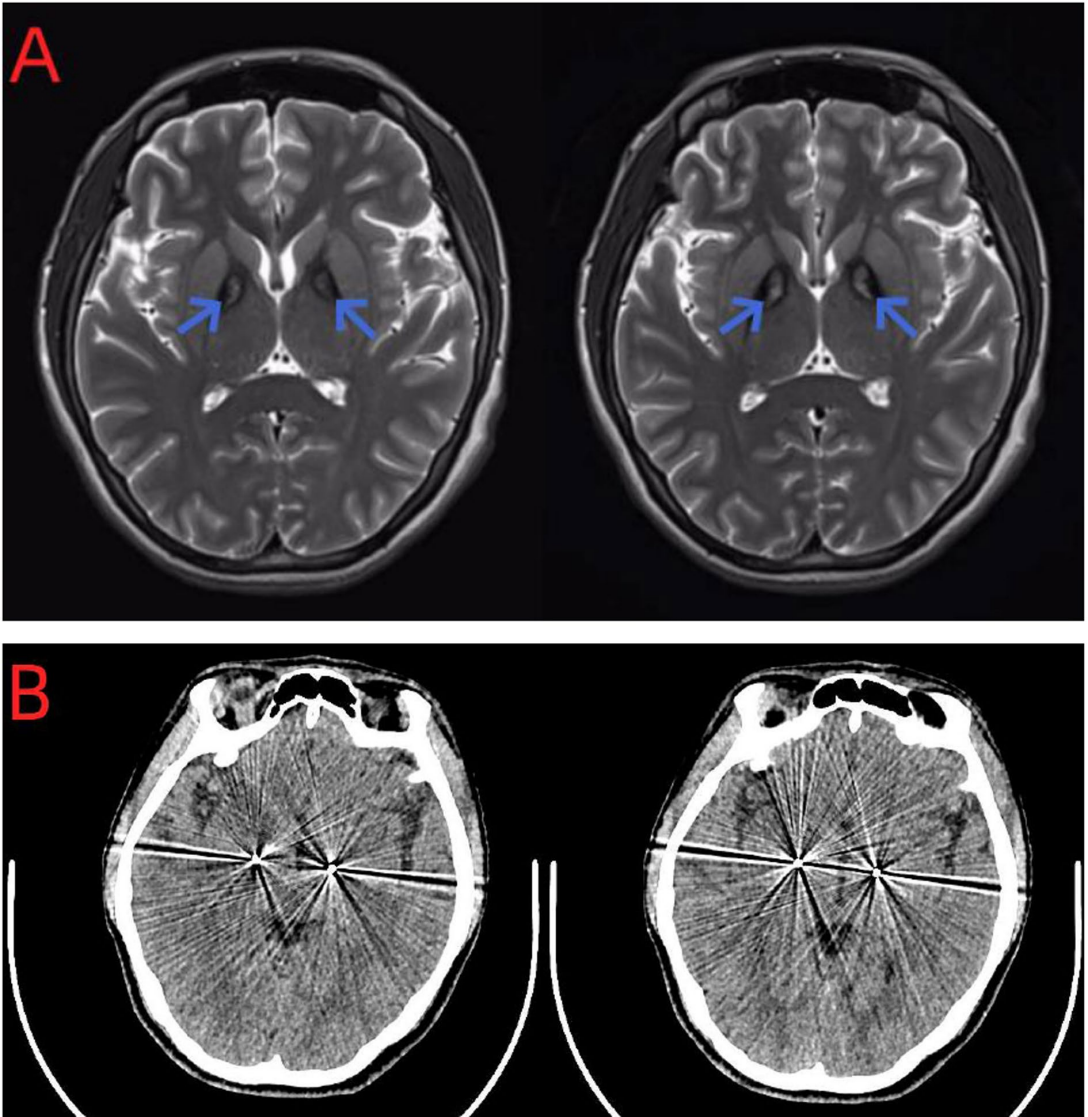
(A) Axial brain MRI of the patient. MRI T2WI showed the “tiger eye” sign. (B) CT showed the implantation site of the permanent DBS electrode (Medtronic, United States).
